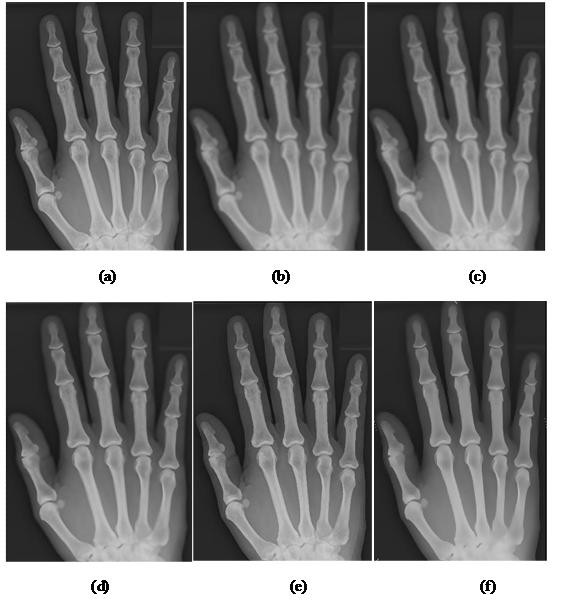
Comparisons of radiograph processed by anisotropic diffusion with various alternatives. In this figure, the radiograph is diffused by different algorithm for comparison: (a) Original image (b) Gaussian filter (c) Average filter (d) Wiener filter (e) Symmetric Nearest Neighbor (SNN) filter (f) Anisotropic diffusion.
